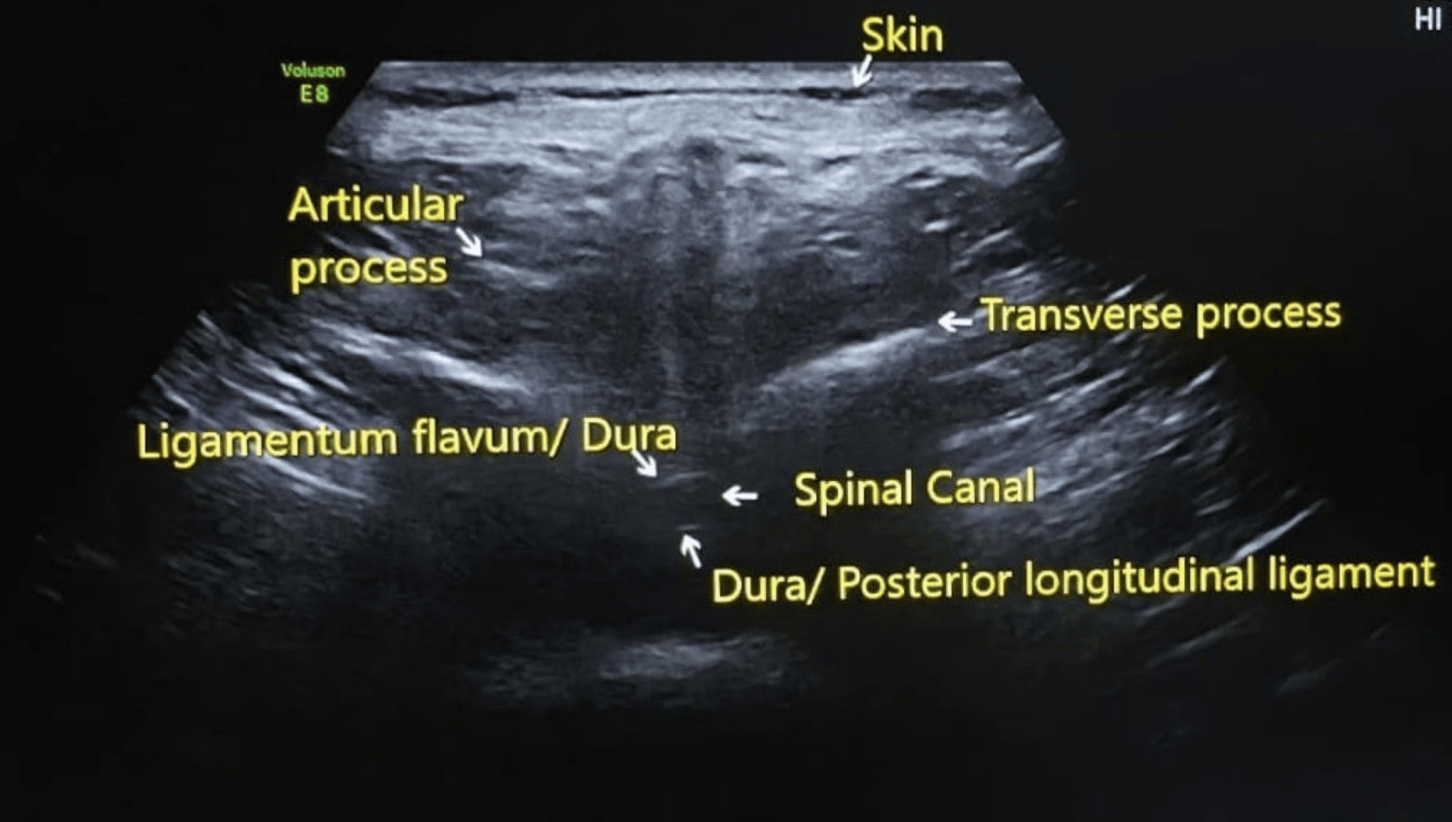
Ultrasound image of L3-L4 spine
